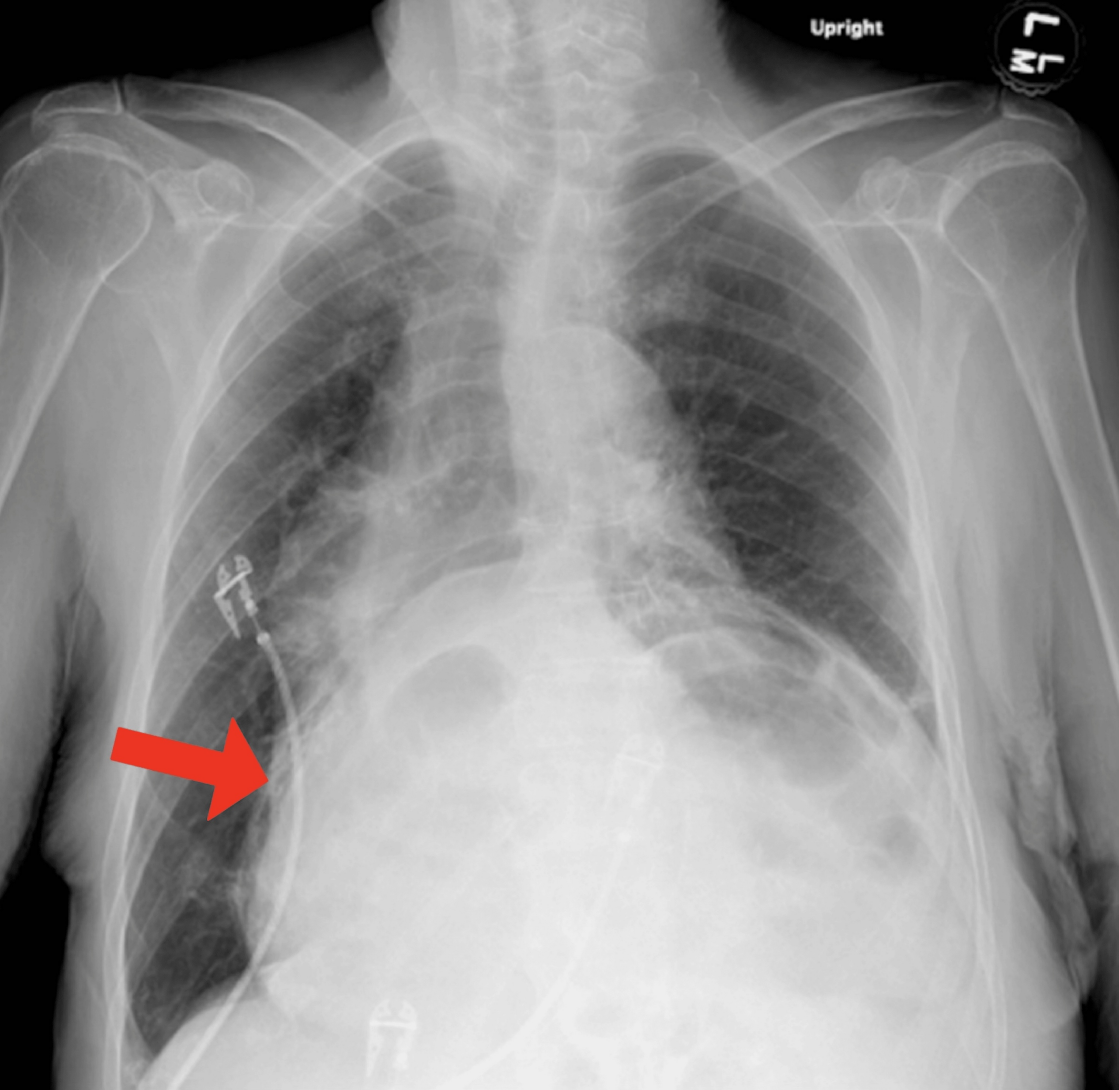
Chest X-ray showing large hiatal hernia sac (red arrow) with mediastinal deviation.
